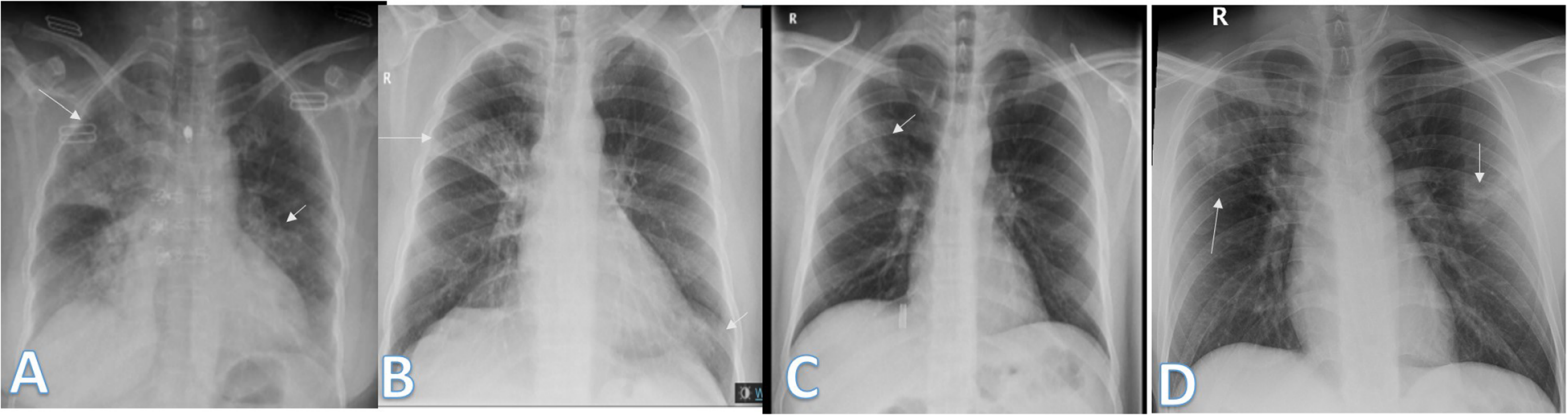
Four different patients with positive COVID-19. a 45-year female was complaining of fever and cough. Initial chest X-ray showed bilateral lower zonal air space consolidation opacities with upper zonal predominance on the right side (long arrow) and perihilar pattern on the left side. The severity score was 2 for the right lung and 1 on the left lung, so TSS was 3. b 60-year male was complaining of fever. Initial chest X-ray showed bilateral air space consolidation opacities with upper zonal predominance on the right side (long arrow) and at the paracardiac area on the left side (short arrow). The severity score was 1 for each lung so, TSS was 2. c 23-year asymptomatic male. Initial chest X-ray showed right upper zonal air space consolidation opacity (arrow). The total severity score was 1. d 28-year male patient presented with cough, on initial X-ray showed right upper zonal (long arrow) and left midzonal (short arrow) air space consolidation opacities; the severity score was 1 for each lung, so TSS was 2
